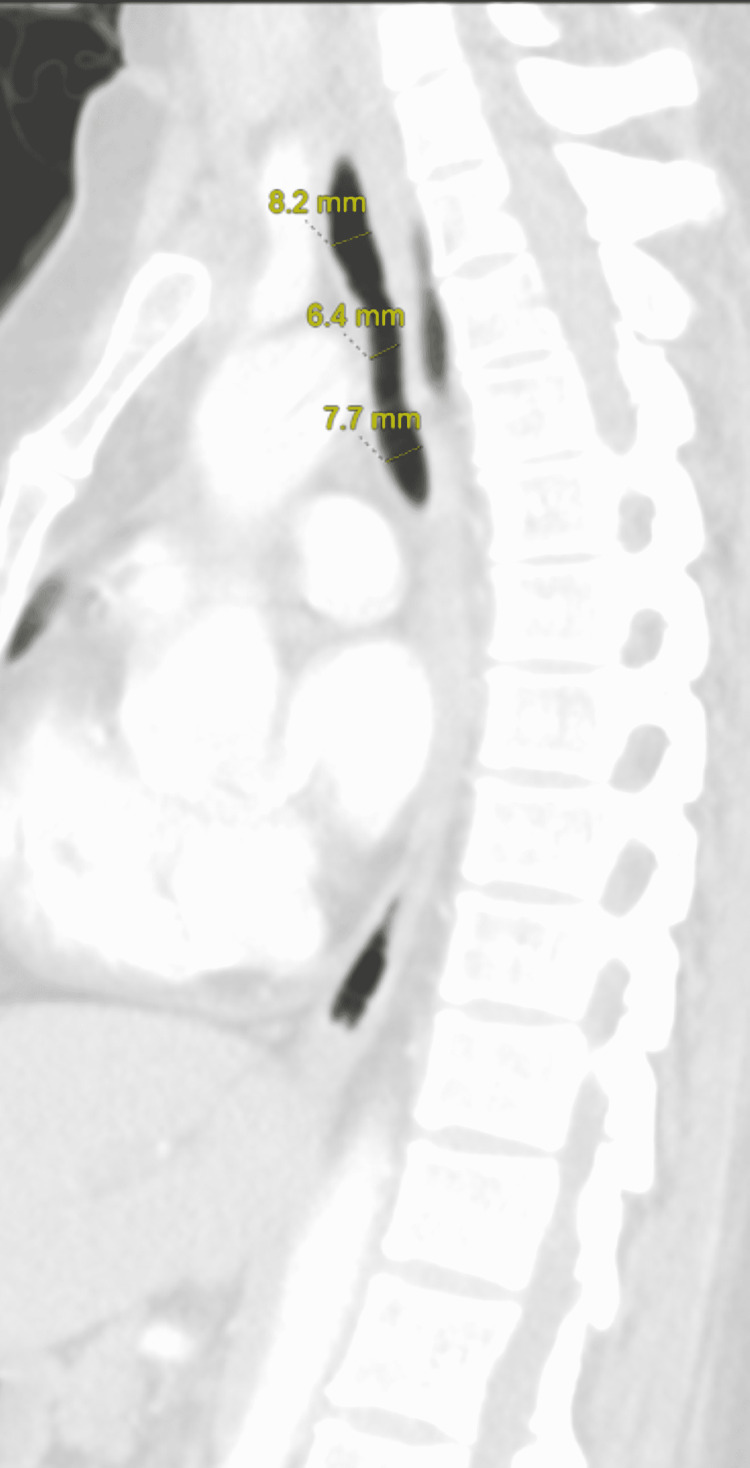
CT Angiogram Pulmonary Embolism with Omnipaque Sagittal view showing distal tracheal stenosis.
